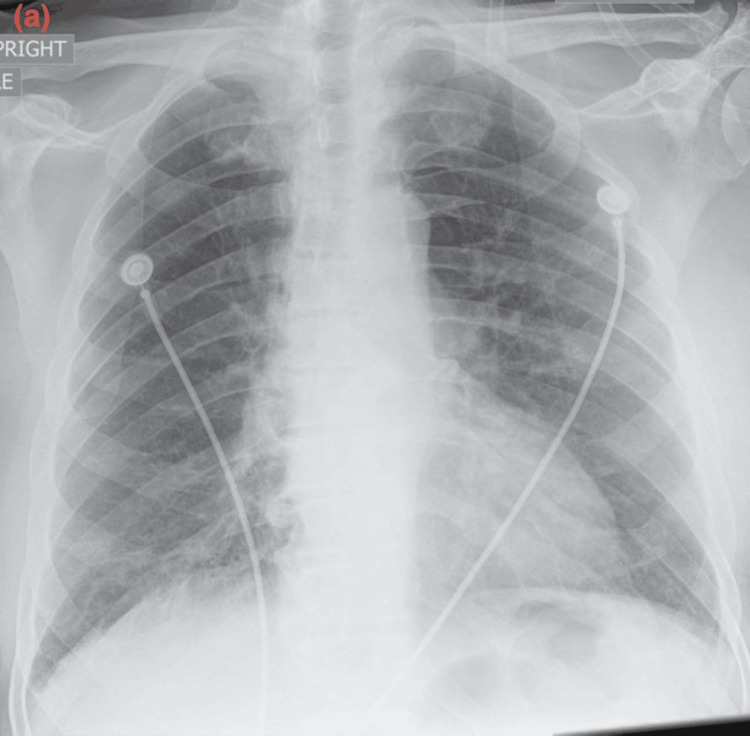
Progression of the disease on chest X-ray: (a) Chest X-ray before starting therapy showing no evidence of acute disease.
